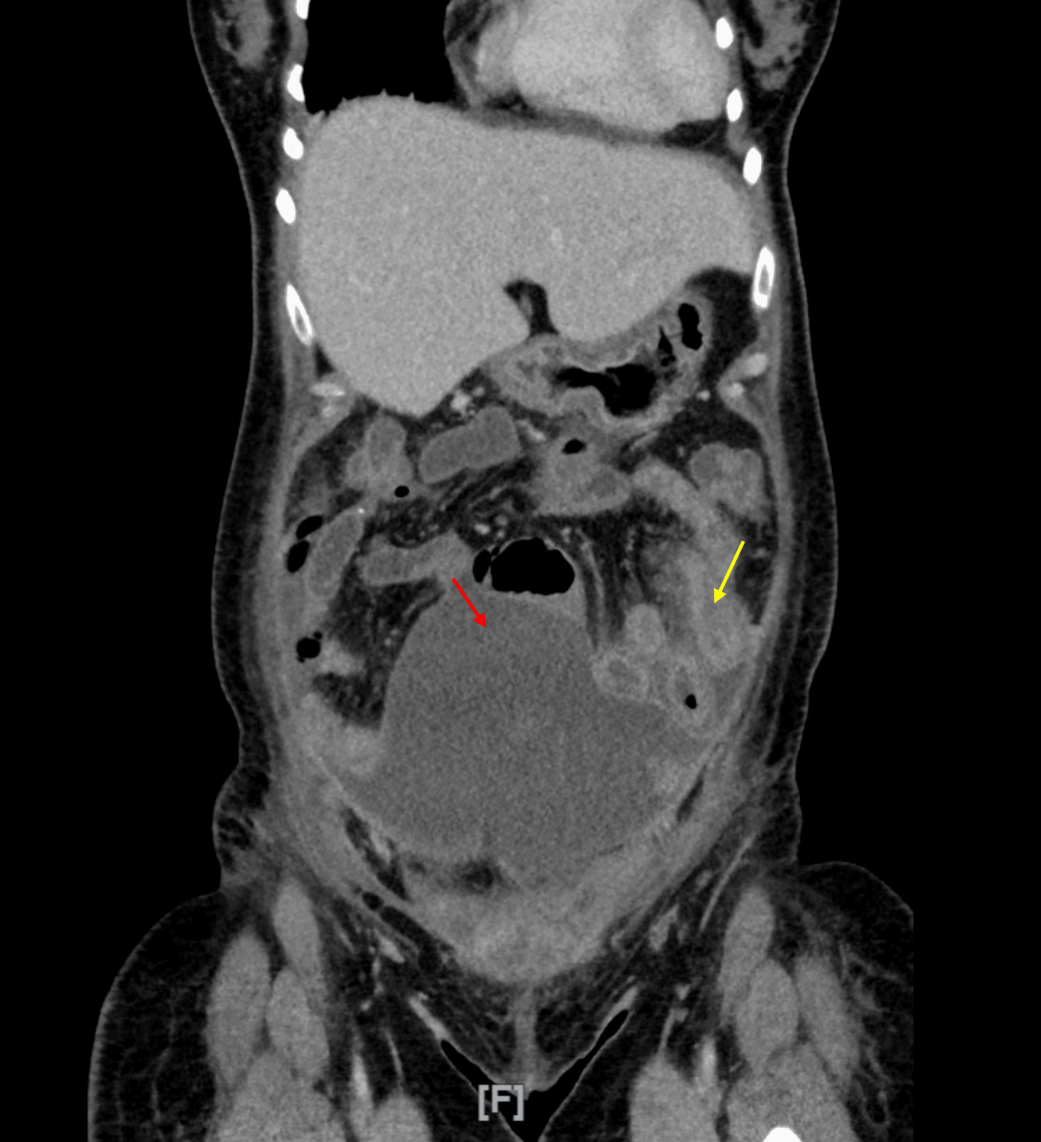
Repeat coronal slice through contrast-enhanced CT abdomen and pelvis from day 6 of admission ⁠Red arrow indicates a large fluid collection superior and anterior to the uterus, measuring 15.8 cm in the craniocaudal dimension and 6 cm in the anteroposterior dimension. The wall of the collection demonstrated contrast enhancement. There was communication with a collection in the parietal wall. Yellow arrow indicates secondary inflammation of the adjacent bowel, demonstrating wall thickening and surrounding fat stranding.
